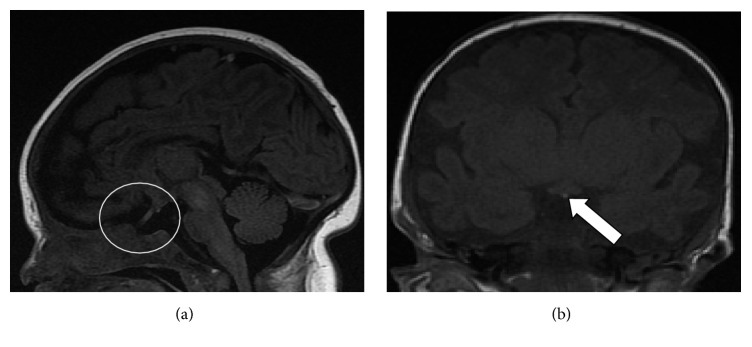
(a) MRI. Sagittal view demonstrates no evidence of the sella turcica and no pituitary soft tissue within the presumed area of the sella. (b) MRI. Coronal view demonstrates a superiorly displaced T1 bright spot consistent with an ectopic posterior pituitary.
